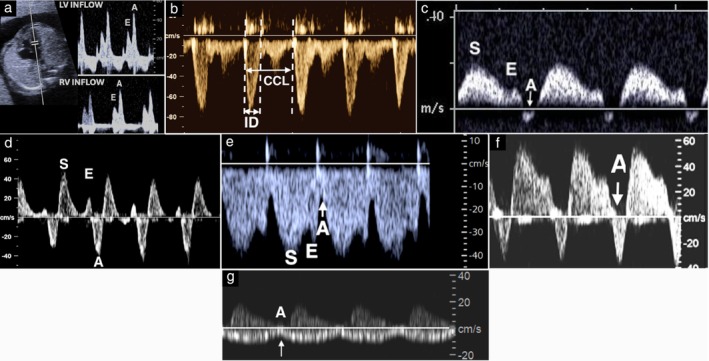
Assessment of diastolic function through pulsed‐wave Doppler interrogation. (a) Normal left (LV) and right (RV) ventricular Doppler inflow acquisition requires alignment of the Doppler sample volume parallel to flow (< 20°), at or just below the atrioventricular valve annulus, as demonstrated for the LV (left panel). Normal Doppler flow profile sampled through the mitral (left) and tricuspid (right) valves demonstrates the usual biphasic flow pattern with E‐ (early diastole) and A‐ (atrial contraction) waves and a duration of nearly half the cardiac cycle length. (b) Uniphasic ventricular inflow (ID, inflow duration) of very short duration (during atrial systole) relative to the cardiac cycle length (CCL) is shown in a fetus with dilated cardiomyopathy. (c) Normal inferior vena cava and hepatic venous Doppler profile demonstrates three phases: forward flow in systole (S), forward flow in early diastole (E) and short velocity A‐wave reversal during atrial contraction (A). (d) With increased ventricular and atrial filling pressures there is loss of flow in early diastole and progressive A‐wave reversal (velocity and duration). Eventually flow may become bidirectional. (e) The normal ductus venosus flow profile has the same three phases as seen for the systemic veins; however, during atrial systole there is a reduction in forward flow velocity and no flow reversal should be present. (f) With increasing central venous pressures due to poor function, there is eventual reversal of flow in the ductus venous during atrial systole. (g) Umbilical venous Doppler demonstrates abnormal pulsatility with reduction or cessation of flow in atrial systole. Normally, there is low velocity non‐phasic flow or undulating flow with fetal breathing.
